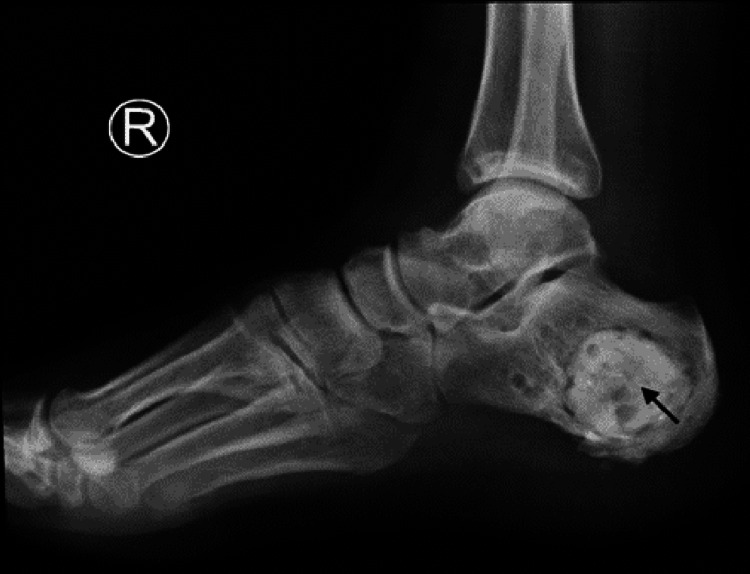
X-ray of the right foot demonstrating bone cement in the right calcaneus (black arrow)
